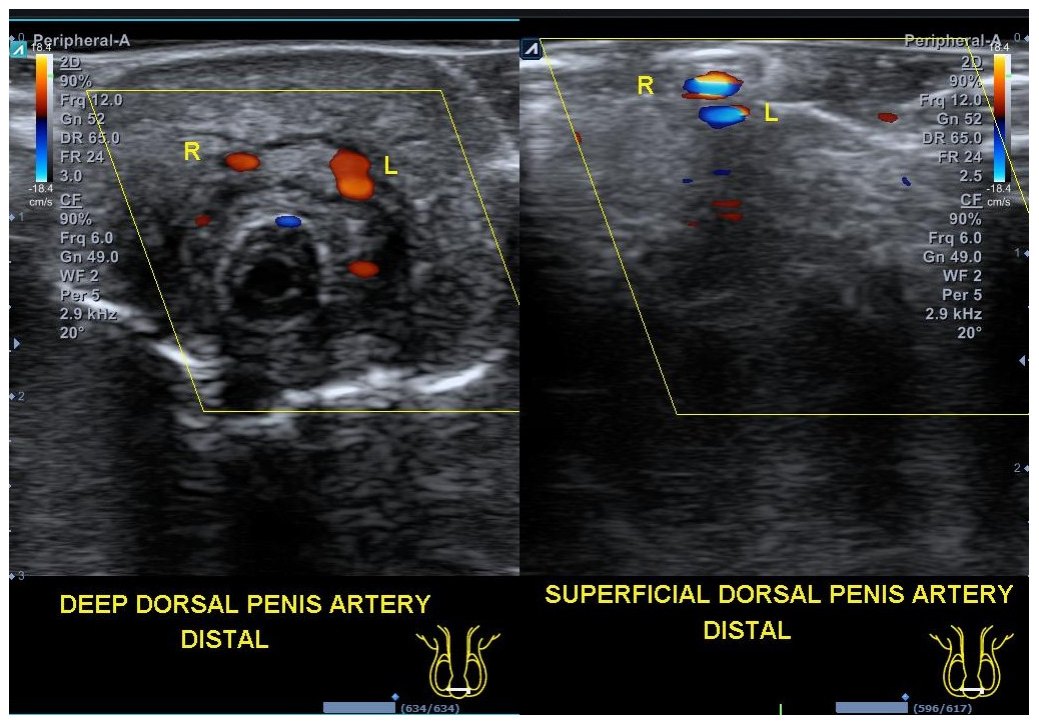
Post-surgical evaluation study at the fifth day with Doppler ultrasound showed adequate deep and superficial arterial flow at the distal end of the penis.
